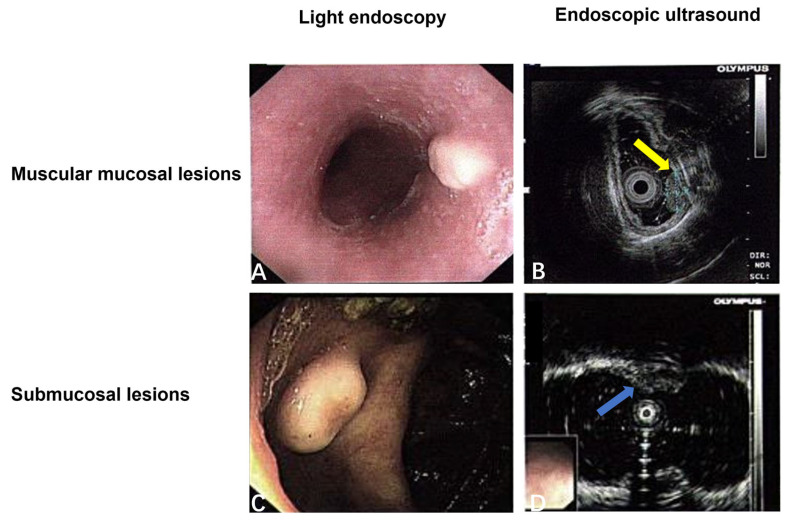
(A,B) Mucosal muscularis lesions under white light endoscopy and endoscopic ultrasound (yellow arrow); (C,D) submucosal lesions under white light endoscopy and endoscopic ultrasound (blue arrow).
